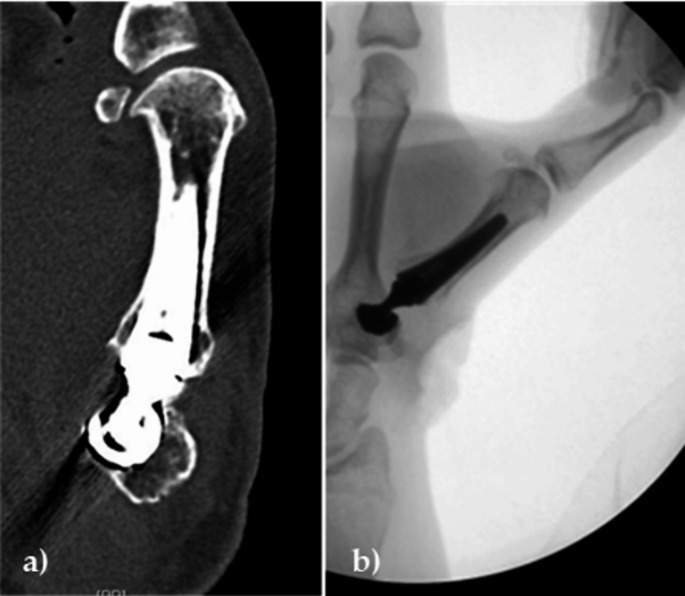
a) Radiographic imaging of a patient suffering from impingement between the neck and cup components, restricted movement shown in CT before revision surgery; b) Free movement of the prosthesis during intraoperative mobilization after replacement of the neck component
